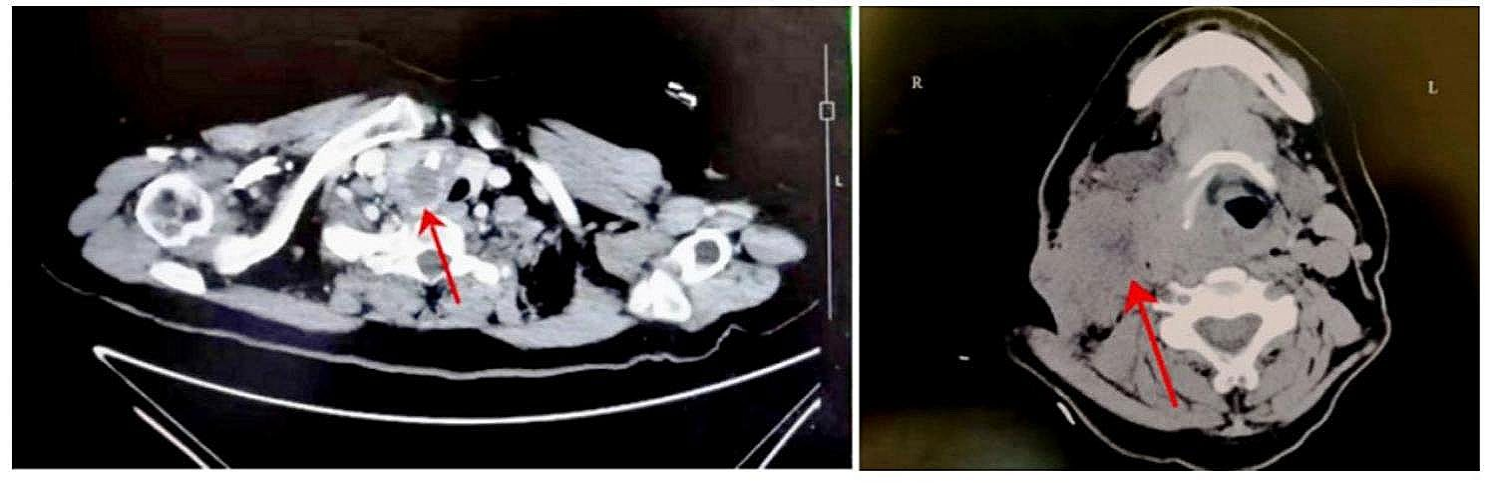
Enhanced CT of the thyroid gland. This picture suggests a malignant tumor right lobe of thyroid gland, with multiple enlarged and fused lymph nodes in the right cervical space
